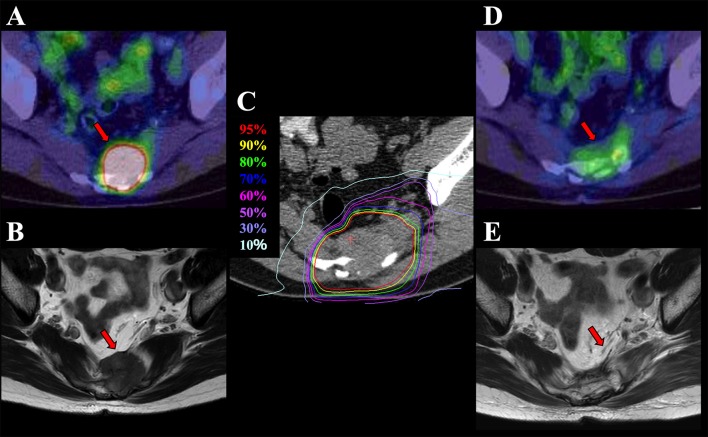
Pelvic recurrence of rectal cancer in a 58-year-old man treated with C-ion RT. (A) PET before treatment. (B) MRI before treatment. (C) Dose distribution on axial CT images. Highlighted are: 95% (red), 90% (yellow), 80% (green), 70% (blue), 60% (pink), 50% (purple), 30% (light purple), and 10% (light blue) isodose curves [100% = 73.6 Gy [RBE]]. (D) PET 3 months after treatment. (E) MRI 12 months after treatment demonstrating disappearance of the presacral mass. Arrows show the recurrent tumor. C-ion RT, carbon-ion radiotherapy; PET, positron emission tomography; MRI, magnetic resonance imaging; CT, computed tomography; RBE, relative biological effectiveness.
